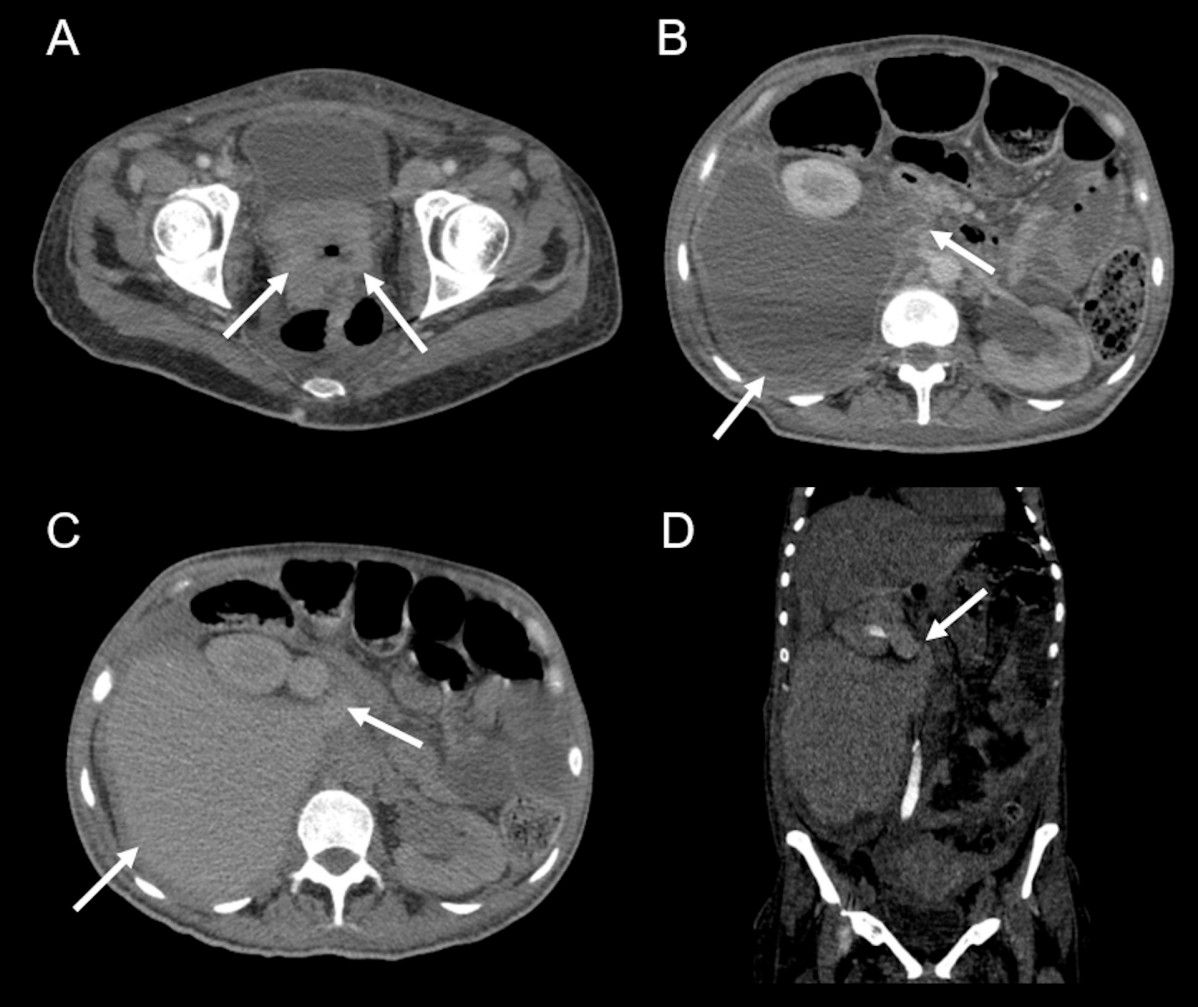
Urinoma due to rupture of the right ureter, secondary to obstruction due to cervical cancer. Computed tomography with iodinated contrast media showed, in the portal phase, thickening of the uterine cervix wall with the contrast-enhancement, invading the urinary bladder trigone (arrows in A), associated with bilateral hydronephrosis and a retroperitoneal fluid collection adjacent to the right ureter (arrows in B). In the excretory phase of the computed tomography, there was extravasation of the contrast media from the right ureter into the fluid collection (arrows in C). Reconstruction in the coronal plane of the exam better demonstrated the communication between the right ureter and the fluid collection, characterizing the urinoma (arrow in D).
